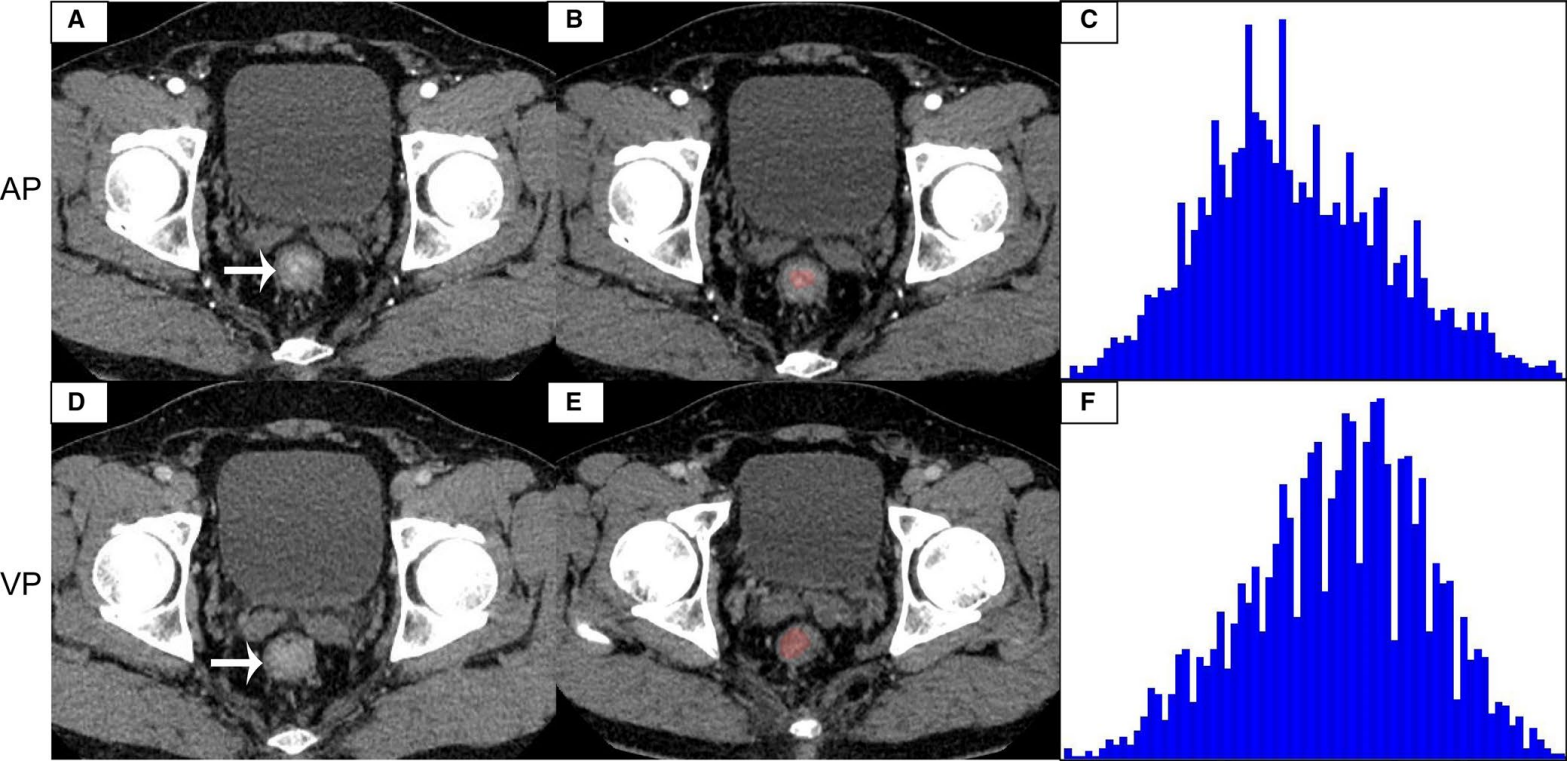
A 51‐year‐old male with G1 R‐NETs. The tumor was significantly enhanced on the arterial phase image (A), and the degree of enhancement is reduced on the venous phase image (D). The tumor region of interest (ROI) was localized on axial CT images at arterial phase (B) and venous phase (E). CT histogram at arterial phase (C) and venous phase (F) were shown
